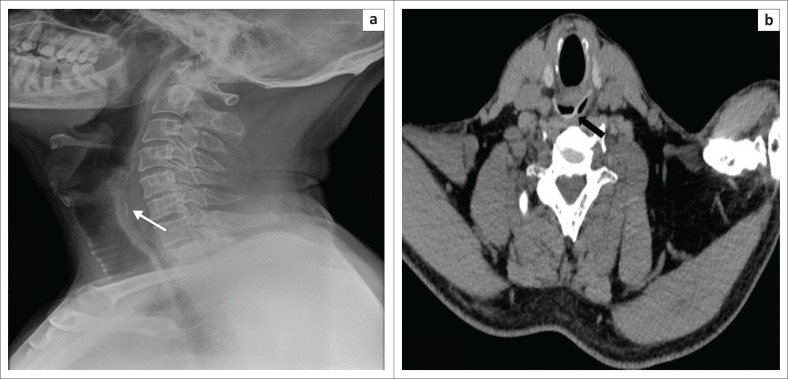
(a) Lateral radiograph of the neck revealing a swallowed denture (thin arrow) at the level of the cricopharynx. (b) Axial computed tomography image confirms the location of the denture (thick arrow), which appears as a curvilinear hyperdense structure.
